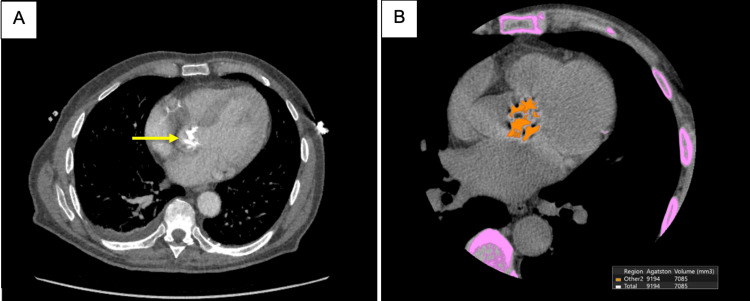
(A) CT coronary angiogram showing a calcified aortic valve (yellow arrow). (B) Calcium scoring demonstrating aortic valve calcification with an Agatston score of 9,194 highlighted in orange. The yellow arrow indicates the calcified aortic valve, while the orange area represents the aortic valve with calcium scoring software applied to calculate the Agatston score. Purple areas represent bone, used as a reference to quantify the degree of calcification.
